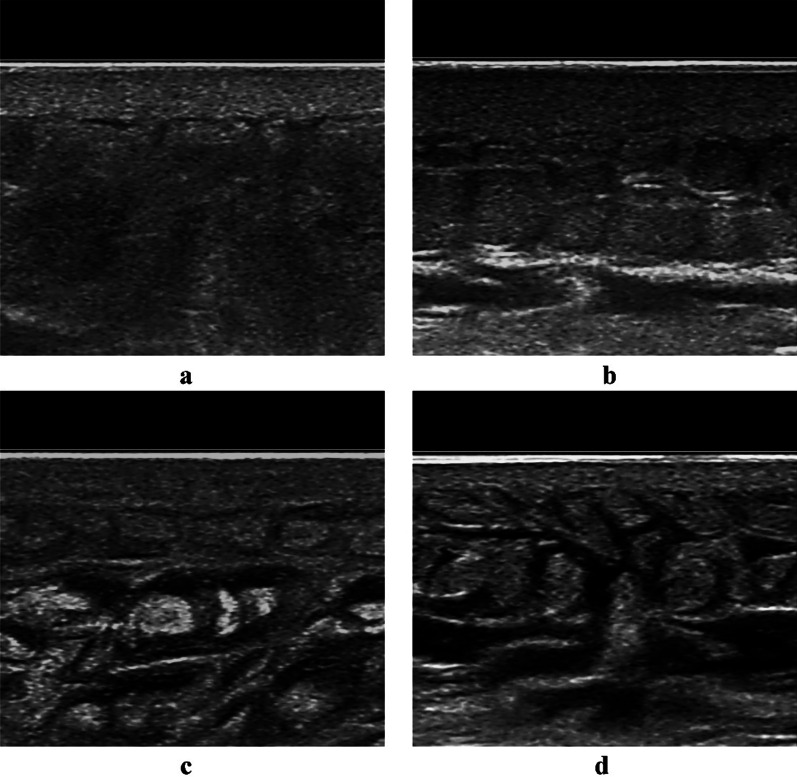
Ultrasonic manifestation of the gravity-dependent features of subcutaneous edema in the abdominal wall. Ultrasonic appearance of subcutaneous edema with Grade 1 in site A1 (a); ultrasonic appearance of subcutaneous edema with Grade 2 in site A2 (b); Ultrasonic appearance of subcutaneous edema with Grade 3 in site A3 (c); ultrasonic appearance of subcutaneous edema with Grade 4 in site A4 (d)
